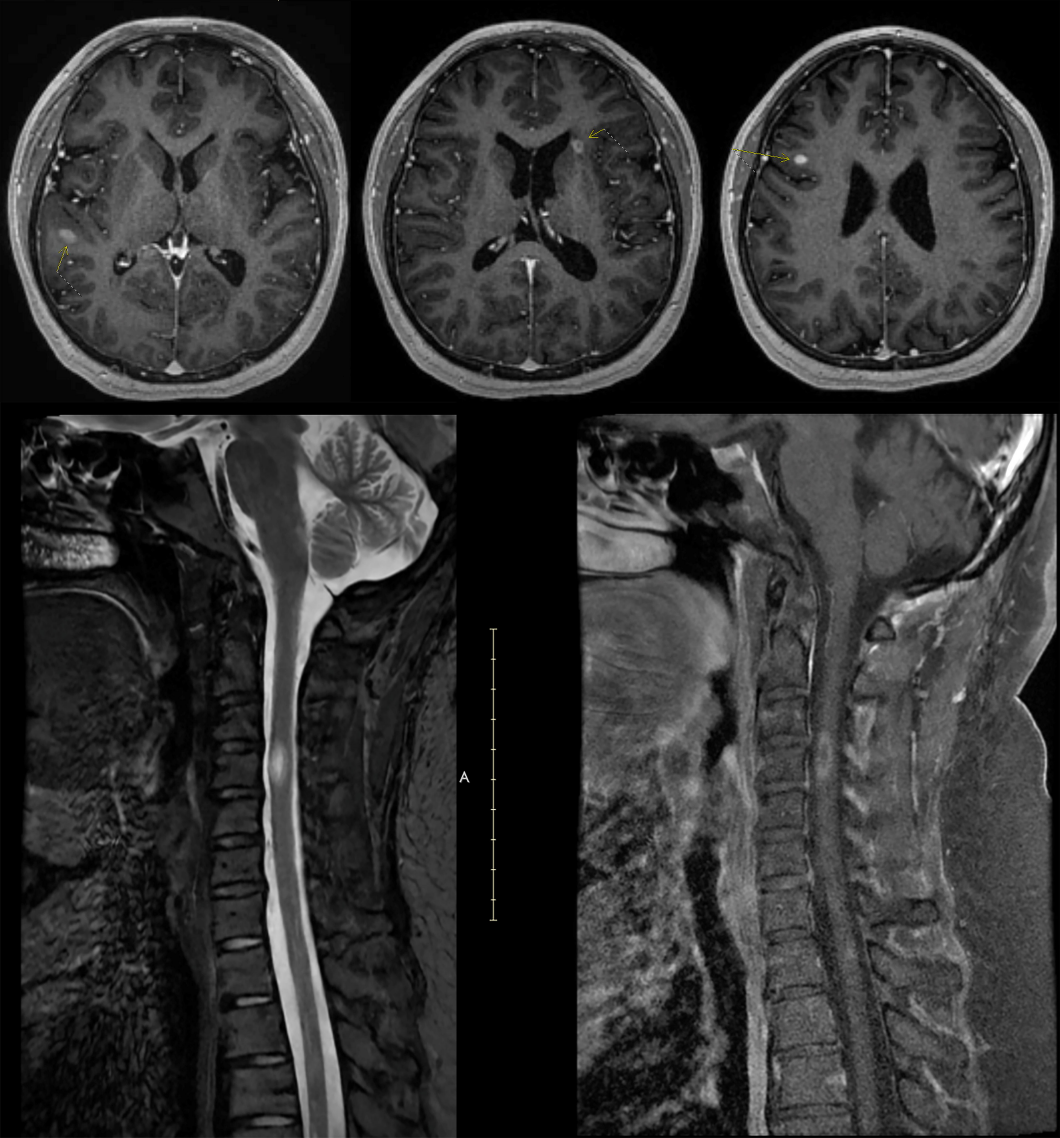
Radiographic images of Case 1 obtained after initiation of ocrelizumab. Cervical MRI obtained 5 months after the seventh cycle of ocrelizumab showing a new gadolinium-enhancing lesion in the cervical cord at C4. MRI, magnetic resonance imaging.
